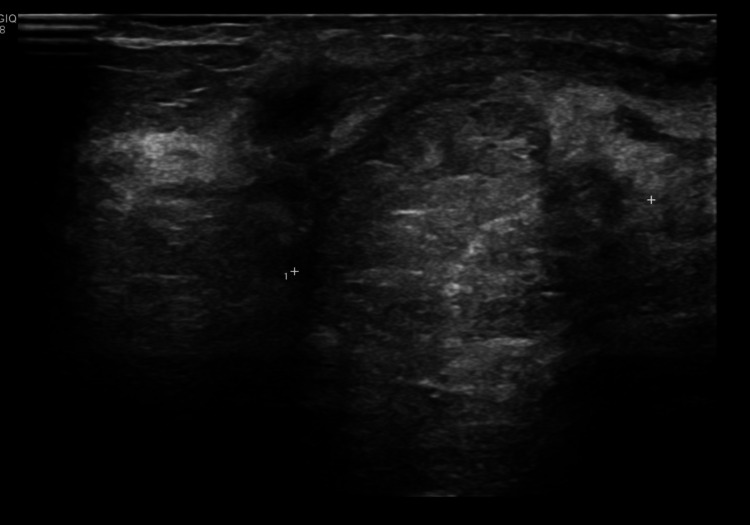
Ultrasound image of the palpable mass prior to biopsy Ultrasound image of the palpable peri-areolar mass at 12 o’clock, mostly isoechoic to surrounding breast tissue, heterogeneous internal echotexture and lobulated contours (biopsy proven invasive pure mucinous carcinoma).
